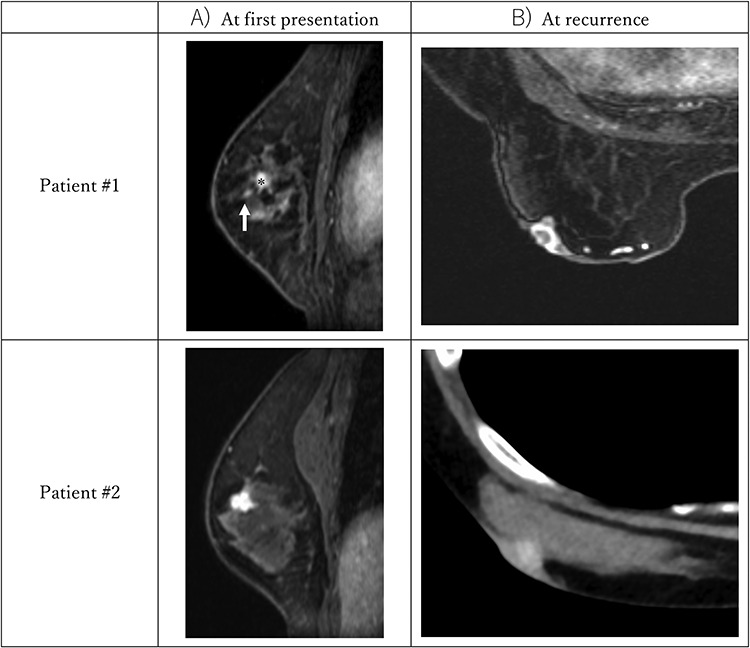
Dynamic contrast-enhanced MRI images of patients #1 and #2 (Table 2) at first presentation and MRI or CT image at recurrence. (A) Sagittal view of delayed-phase (~5 min after gadolinium injection) T1-weighted image (T1WI) of primary tumor at first presentation. Patient #1: a small enhanced nodule (↑) is captured in the nipple direction which indicates the EIC of the primary tumor (^*^). Patient #2: a 1.2 cm enhanced tumor is captured. In this view, the EIC is not completely clear, but as shown in Fig. 2, the axial view is suspected of having EIC. (B) Delayed-phase T1WI (achieved in the prone position) of patient #1 at recurrence and contrast enhanced CT image (achieved in the supine position) of patient #2 at recurrence. Enhanced tumor just under the nipple is captured in both patients.
